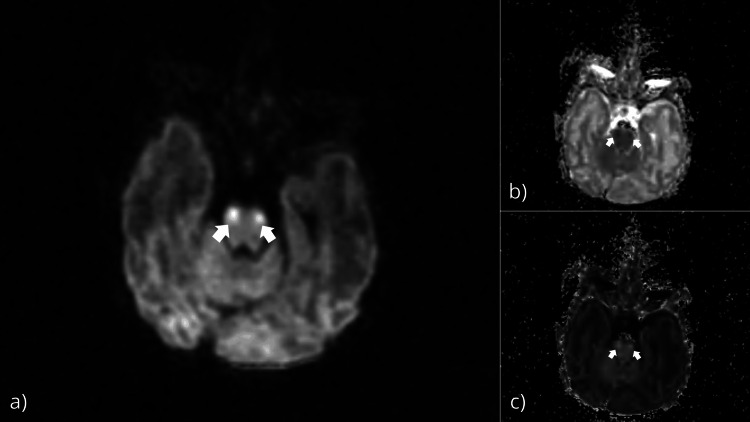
Images of MRI performed at 1 month of age showing ischemic lesions of the brainstem (white arrows) in Axial DWI (a), ADC (b), and e-ADC (c). DWI: diffusion-weighted imaging; ADC: apparent diffusion coefficient
